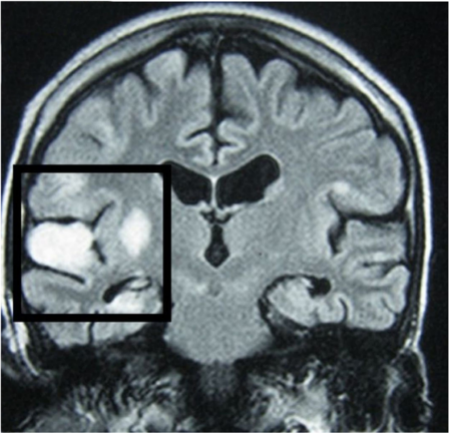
Magnetic resonance image: coronal section of the brain 1 month after the onset of illness. This section shows the involvement of the right cerebral cortex and hippocampus due to multiple cerebral and basal ganglia infarctions
